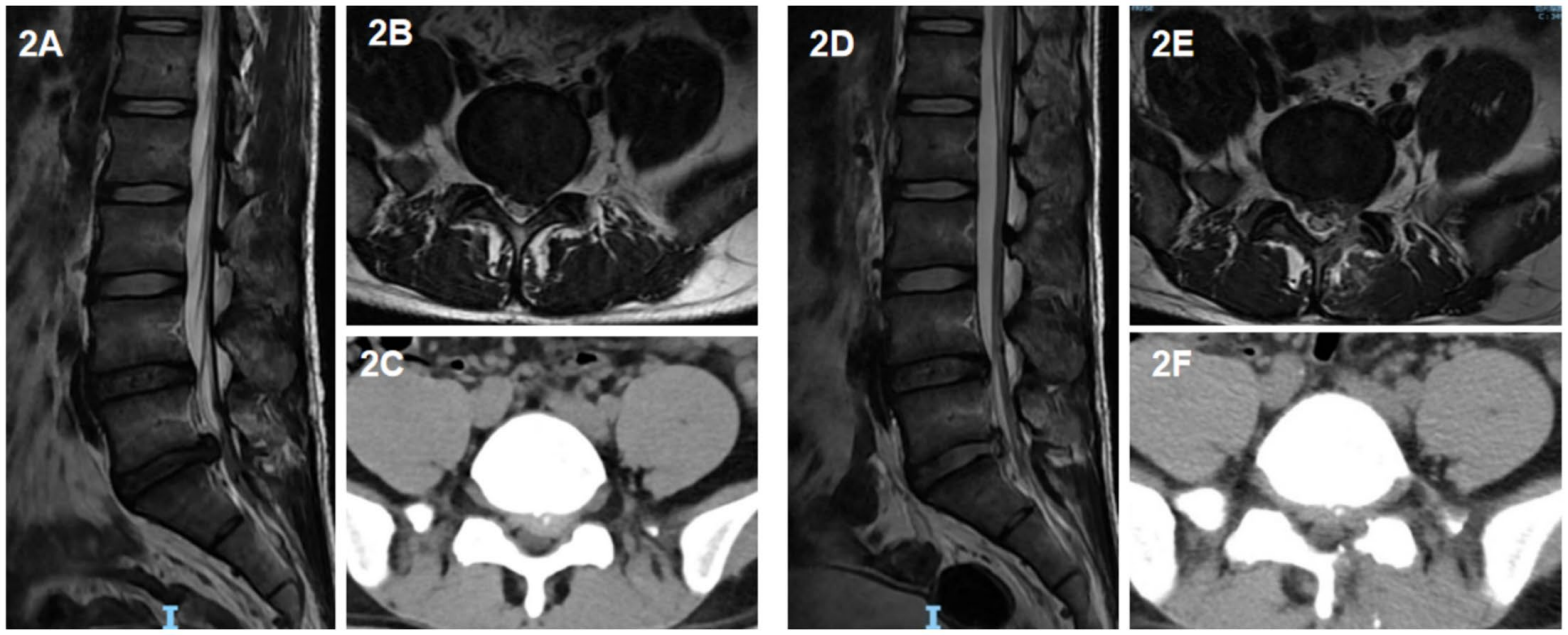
A 39-year-old male patient with left-sided protrusion of the L5/S1 intervertebral disc who underwent UBE surgery. (A) Sagittal view of preoperative lumbar MRI; (B) horizontal view of preoperative lumbar MRI; (C) Horizontal view of preoperative lumbar CT; (D) sagittal view of postoperative lumbar MRI; (E) horizontal view of postoperative lumbar MRI; (F) horizontal view of postoperative lumbar CT.
